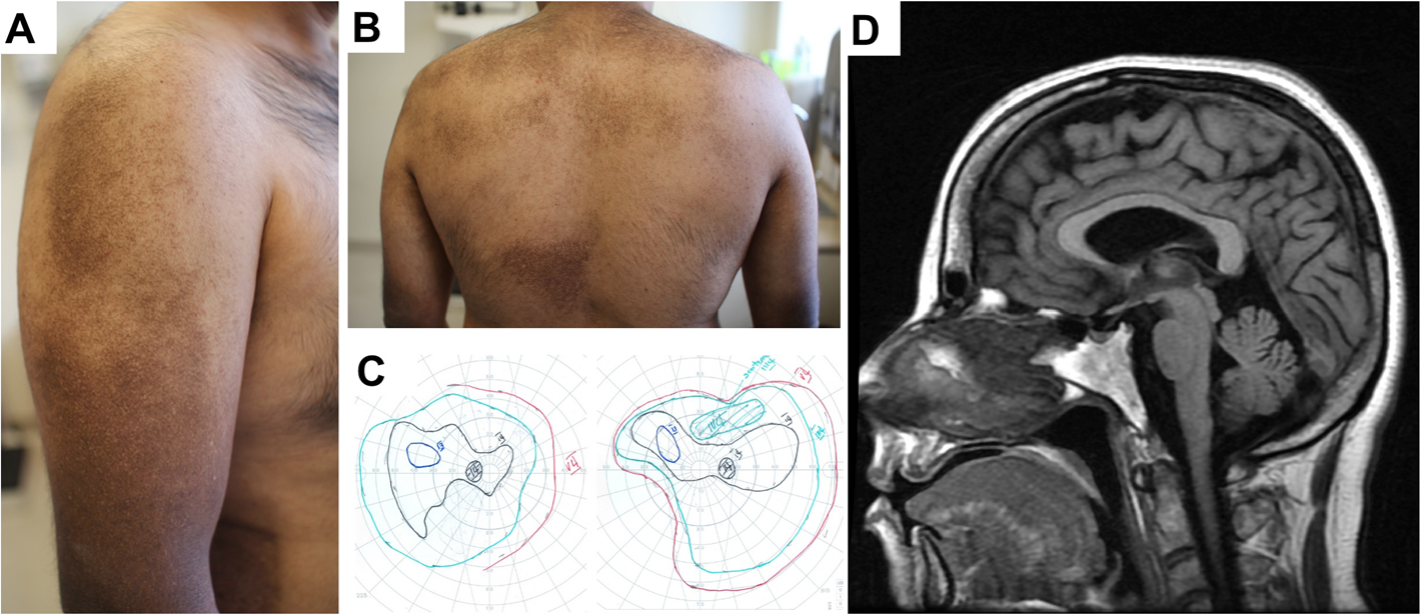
(A) and (B) Macroscopic skin appearances of Brother 2 at age 33 years. He reported hair graying dating from adolescence. He recalled abnormal skin pigmentation appearing in early adulthood; this is in contrast to his sister, whose parents report had developed pigmentary abnormalities in early childhood. Her skin changed in appearance over time, initially exhibiting generalized hyperpigmentation, but with development of hypopigmented macules in early adulthood. (C) Goldmann visual fields of Brother 1 at aged 38 years. Despite significant central and peripheral field loss, he reported no subjective deficits in peripheral vision. His affected sister and brother also were found to have central field loss on visual field testing. Although retinal appearances were grossly normal, retinal visualization with ocular coherence tomography (OCT) showed nerve fiber thinning to varying extents in the three affected siblings. (D) Sagittal T1 MRI study the brain of the female proband at 40 years of age showing mild cerebral and cerebellar volume loss. Subtle white matter signal abnormality in periventricular white matter was also seen on T2/FLAIR sequences (not shown).
